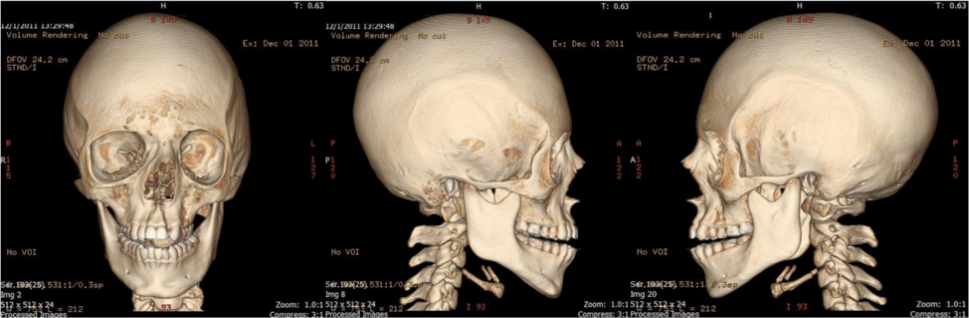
Preoperative frontal, right lateral, and left lateral three-dimensional computed tomography (CT) scans
